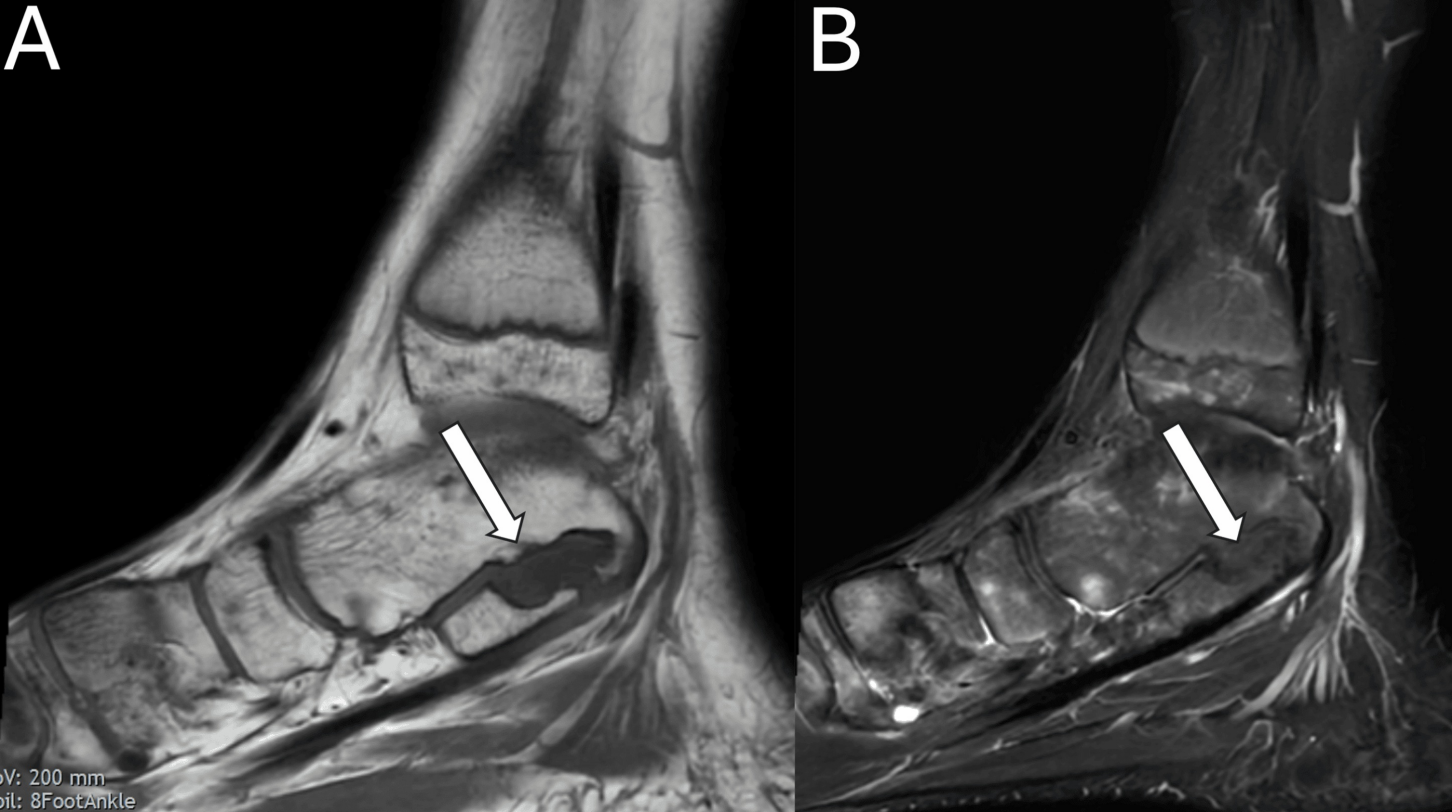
12-month post-operative sagittal (A) T1- and (B) T2-weighted slices of the left ankle without contrast showing RegJoint™ marked with a white arrow.
